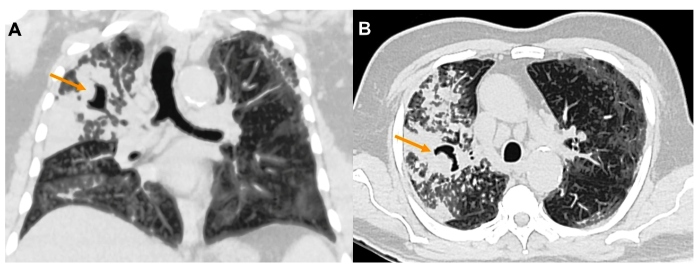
Chest CT in lung window, coronal (A) and axial
